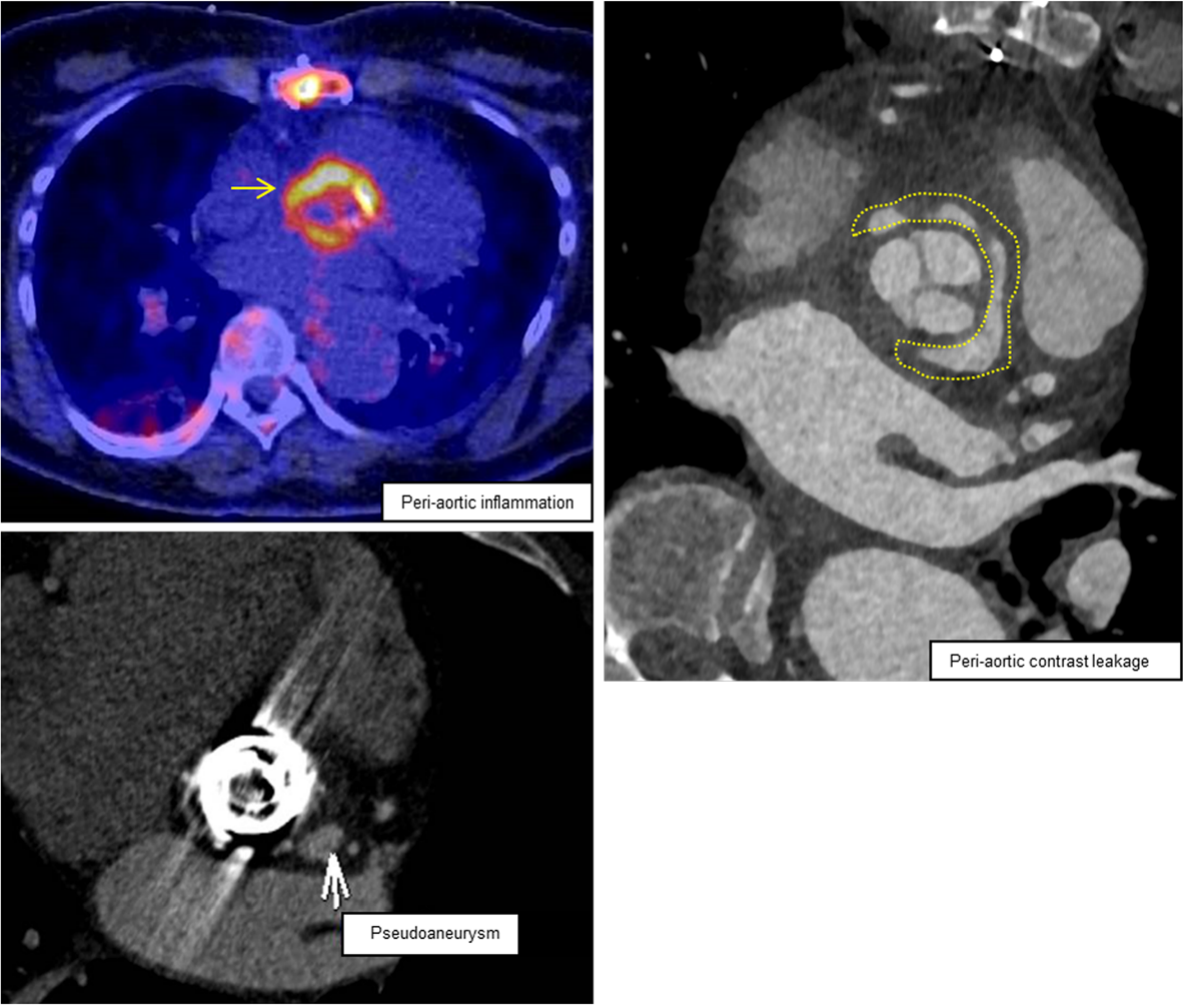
Aortic valve endocarditis with paravalvular abscess formation, nuclear/radiological view with ^18^F-fluorodeoxyglucose positron emission tomography/computed tomography
